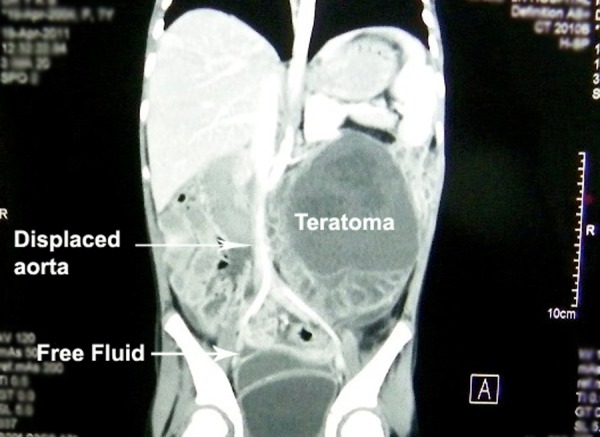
Figure 3: Abdominal CT scan showing well circumcised mass occupying left half of the abdomen, having septations and calcifications and displacing gut and major vessels.
